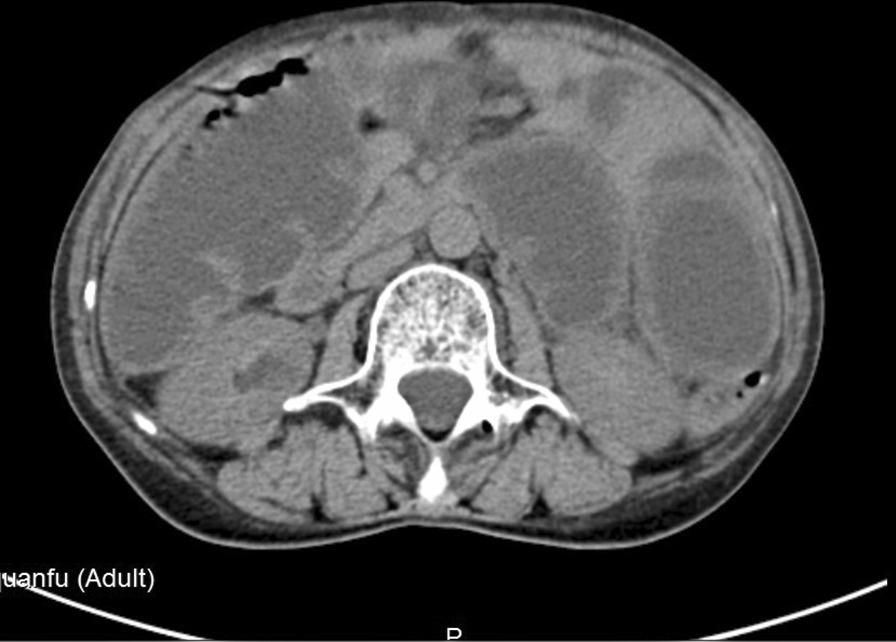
CT of the abdomen reveals incomplete colonic obstruction
